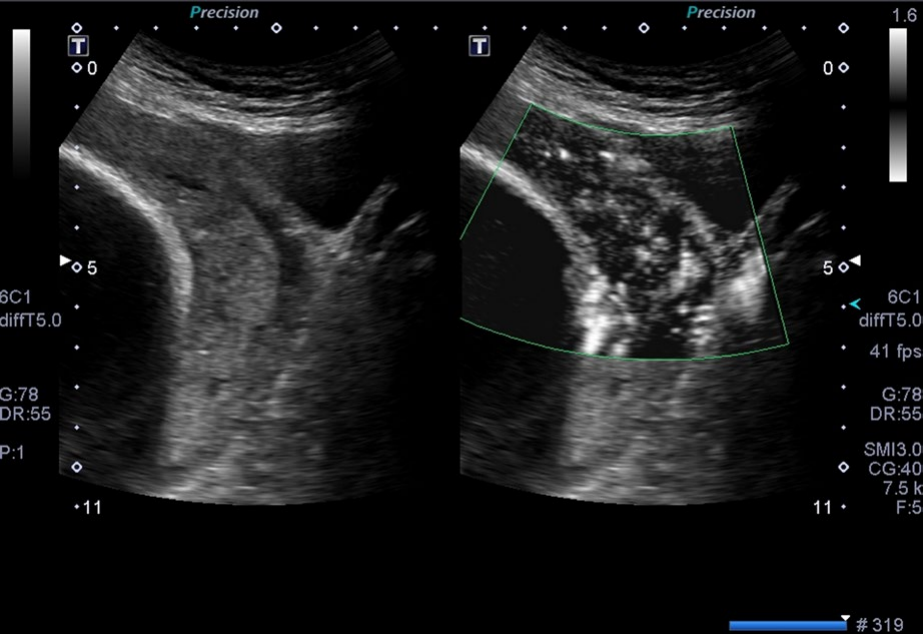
On Sagittal transvaginal ultrasound image placenta is seen extending to the serosal surface of the bladder
